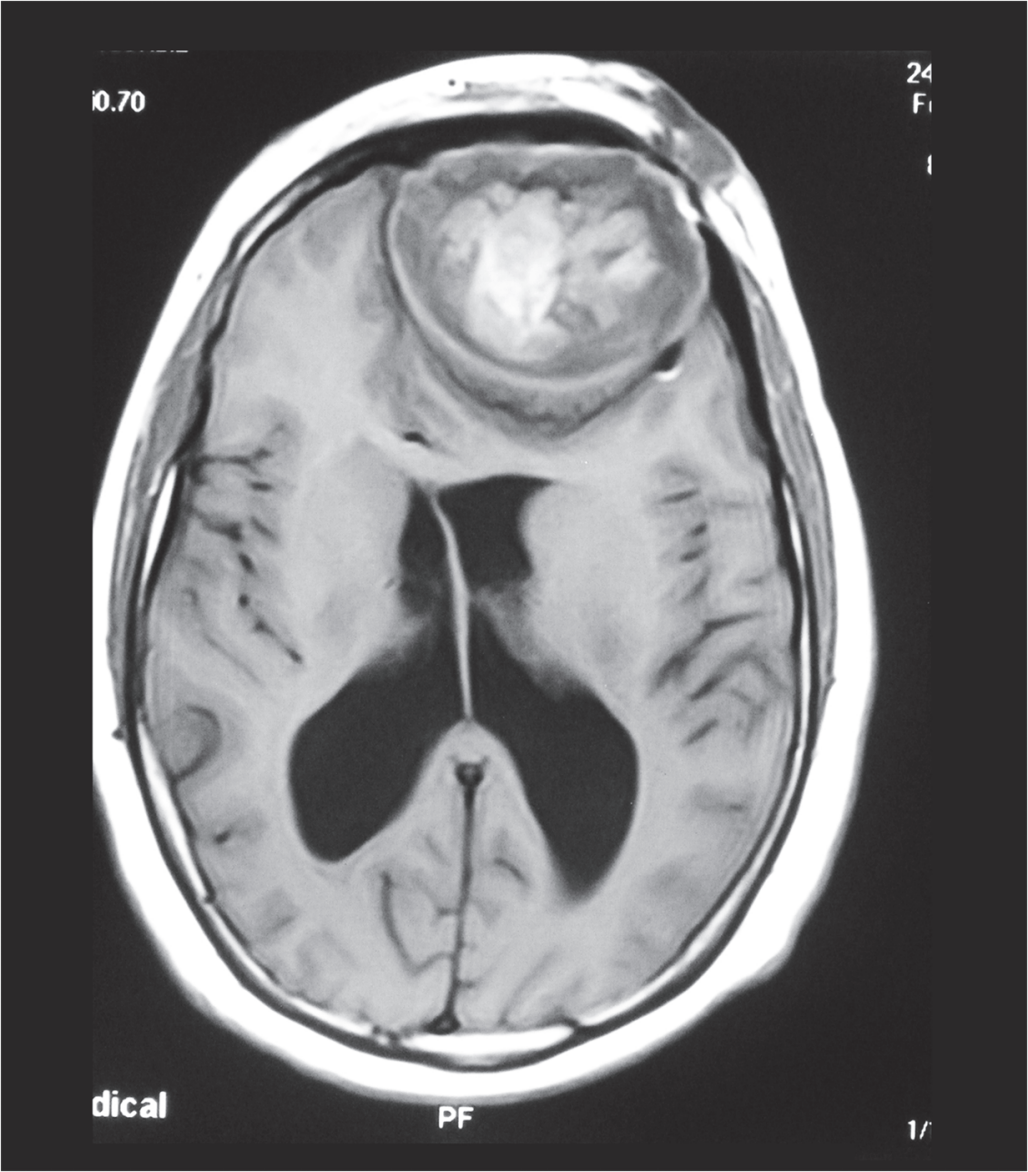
Cranial MRI showing eumycetoma involving the skin, subcutaneous tissue and destruction of the frontal bone and with well encapsulated intracranial lesion.
